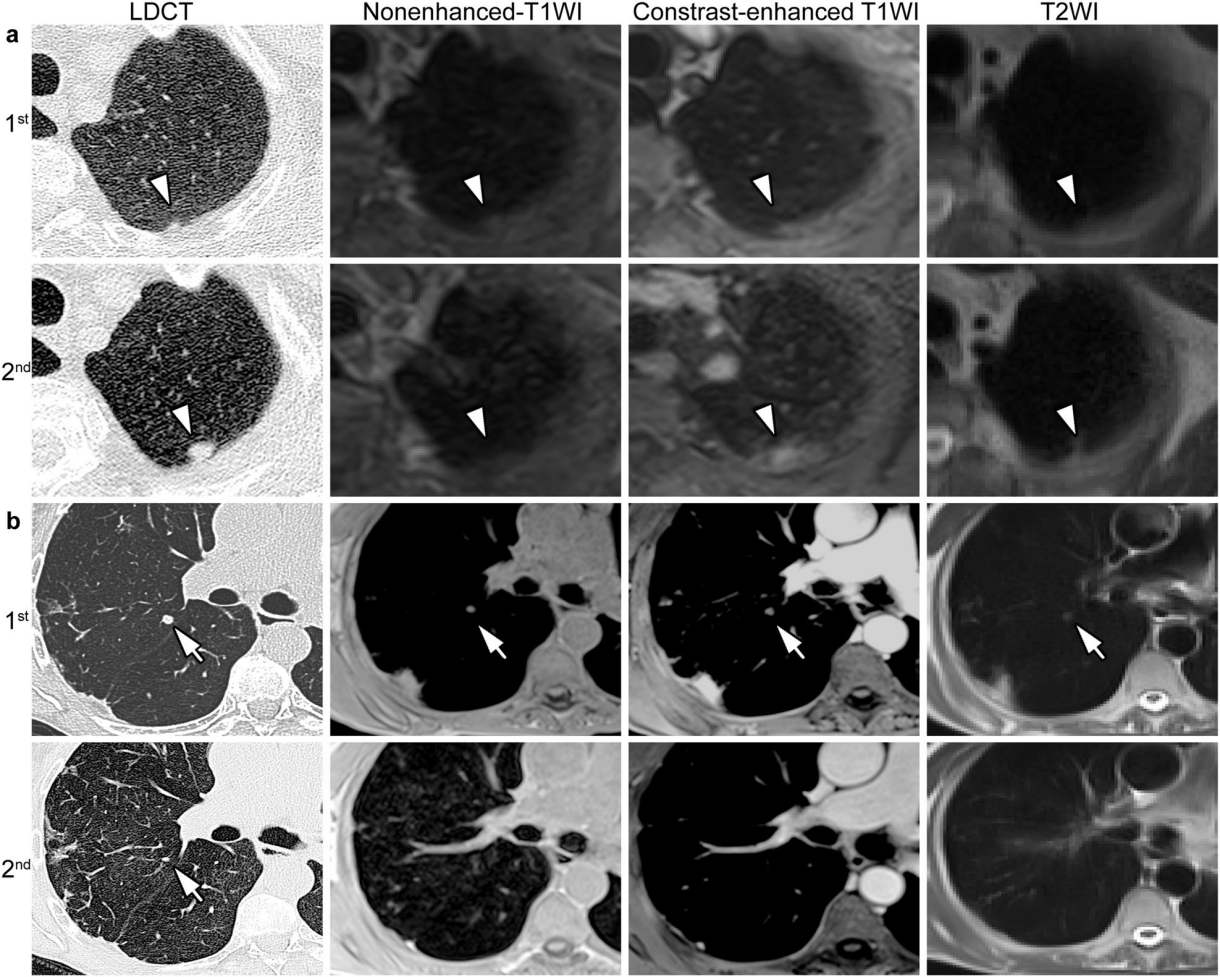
Representative images of progressed and regressed incidental nodules at morpho-functional MRI and LDCT. a A solid pulmonary nodule beside the visceral pleura showed significant growth on the second examination (second row, 2018) compared to the first examination (first row, 2015). The growth can be best displayed on the contrast-enhanced T1-weighted MR images (third column) than the nonenhanced (second column) and T2-weighted images (fourth column). b A solid nodule adjacent to the interlobar pleura was significantly shrunk on the second examination (fourth row) compared to the first round examination (third row). The shrunk nodules on the second imaging round were not displayed on the three MRI sequences
